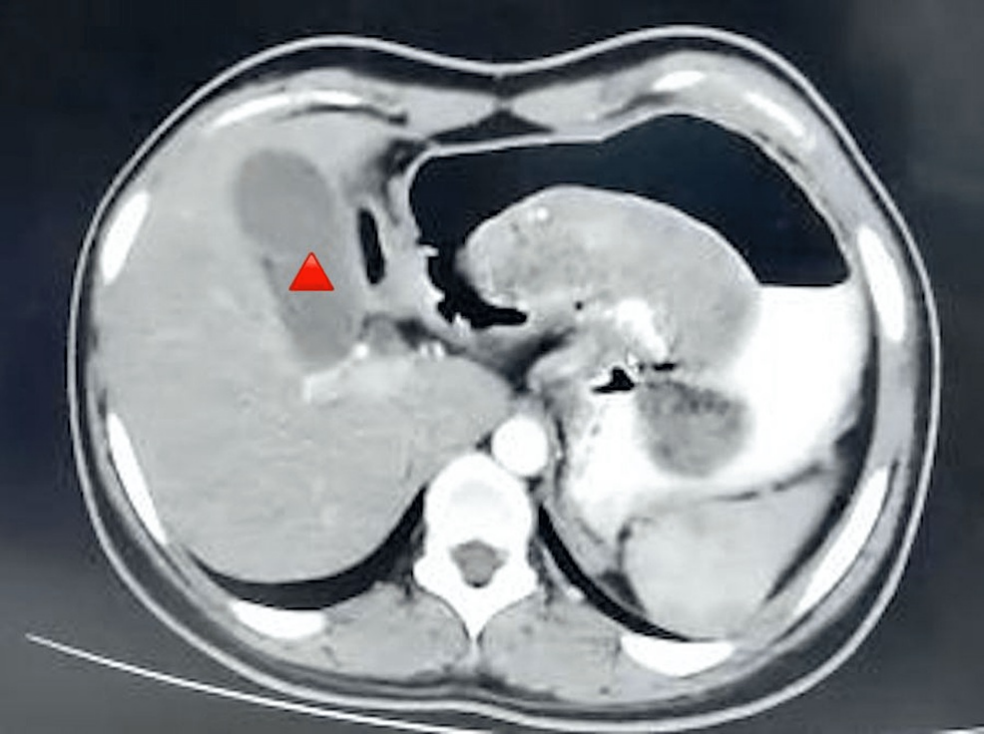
Distension of Stomach With Trichobezoar, CT image Contrast-enhanced CT of the abdomen showed a distended stomach with the bezoar
